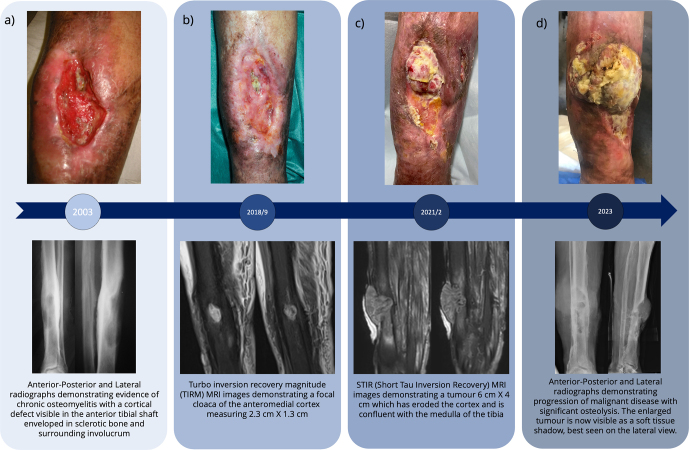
Clinical photographs of ulcer along with radiographs and MRI
